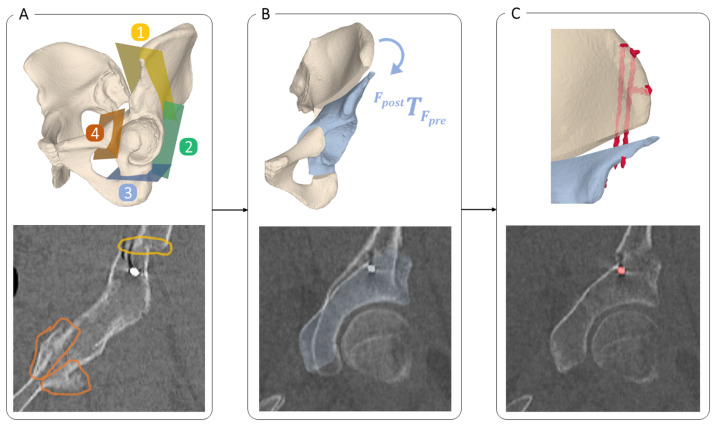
Overview of the three common surgical steps in orthopaedic interventions on the example of the Periacetabular Osteotomy (PAO): (A) Cutting Bone: Four osteotomies are performed, namely the ischial (1), pubic (2), supra- (3) and retroacetabular (4) osteotomy, to mobilise the acetabular fragment (in blue). (B) Anatomy Manipulation and Repositioning: Repositioning of the acetabular fragment (in blue) to restore the physiological anatomy. The transformation ${}^{F_{post}}T_{F_{pre}}$ represents the relative repositioning of the fragment from $F_{pre}$ to $F_{post}$, where $F_{pre}$ and $F_{post}$ are the respective local coordinate systems of the cropped pre- and postoperative CT images. (C) Implant Placement: Fixing the acetabular fragment in its new position using screw implants (red). The bottom row shows slices of a postoperative CT: In (A) the supraacetabular (in yellow) and pubic (in orange) osteotomies are highlighted. An overlap of pre- and postoperative CTs is shown in (B), to indicate the acetabular transformation. Finally, in (C), the cross section of a screw is visible in red.
